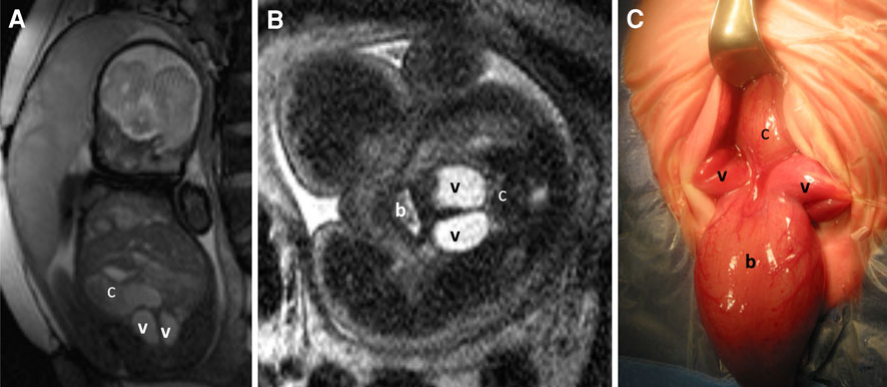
Prenatal MRI of a patient with cloaca. a Coronal view showing bilateral hydrocolpos (*c* colon, *v* vagina). b Axial view (*b* bladder, *v* vagina, *c* colon with calcification). c Operative picture (*b* bladder, *v* vagina, *c* colon)
